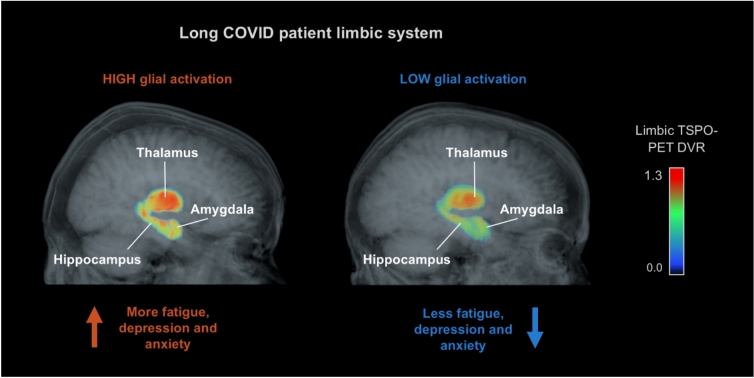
Fatigue, depression and anxiety correlate with limbic TSPO availability in LC participants. The figure shows glial activation in the limbic system of a female LC participant with high limbic TSPO availability (left) and a female LC participant with low limbic TSPO availability (right). Volumetric T1 MRI (grayscale) and limbic TSPO PET (colour gradient) images are overlaid and rendered with transparency to visualise TSPO availability throughout the limbic volume.
